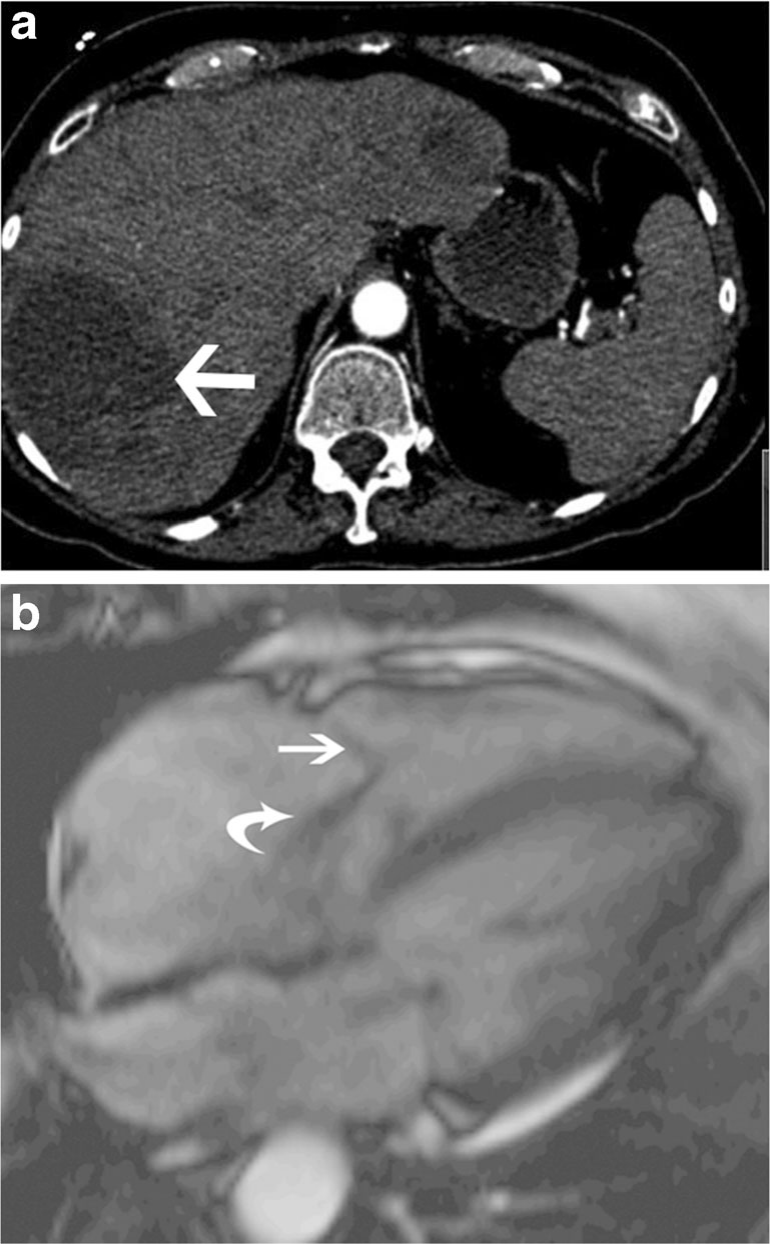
Carcinoid. (a) Axial CT of the abdomen shows a metastatic carcinoid tumour (*arrow*) in the liver. (b) Four-chamber steady-state free precession (SSFP) image shows a thickened tricuspid leaflet (*straight arrow*) with moderate tricuspid regurgitation (*curved arrow*). Mitral regurgitation is also visible
